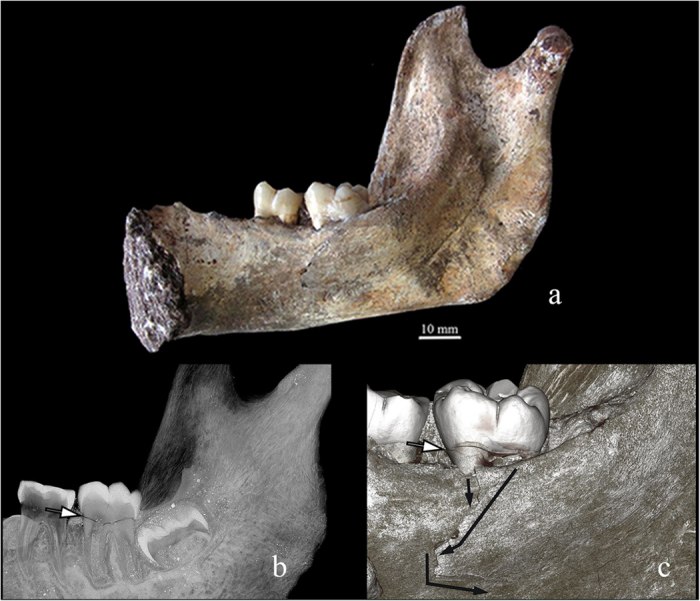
Class I fracture diagnostic of blunt force injury noted on the internal mandible and dentin of the right second lower molar of MH1: (a) overall image of the entire surface of the internal mandible, (b) computed tomography image, (c) vertical cross-section, which displays the fracture traversing through the entire crown.
